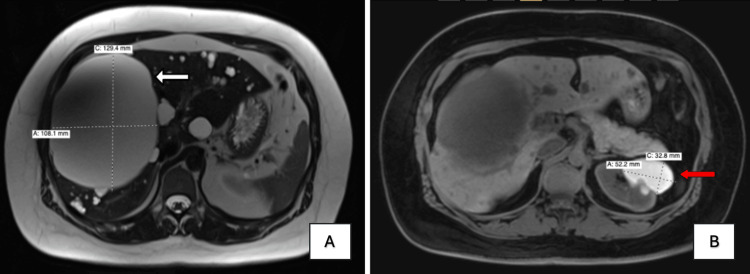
T2-weighted MRI of the abdomen with and without contrast showing hepatic and renal cysts. The white arrow in Figure 1A indicates a 12 × 10 cm hepatic cyst. The red arrow in Figure 1B indicates a 5 × 3 cm left renal cyst.
